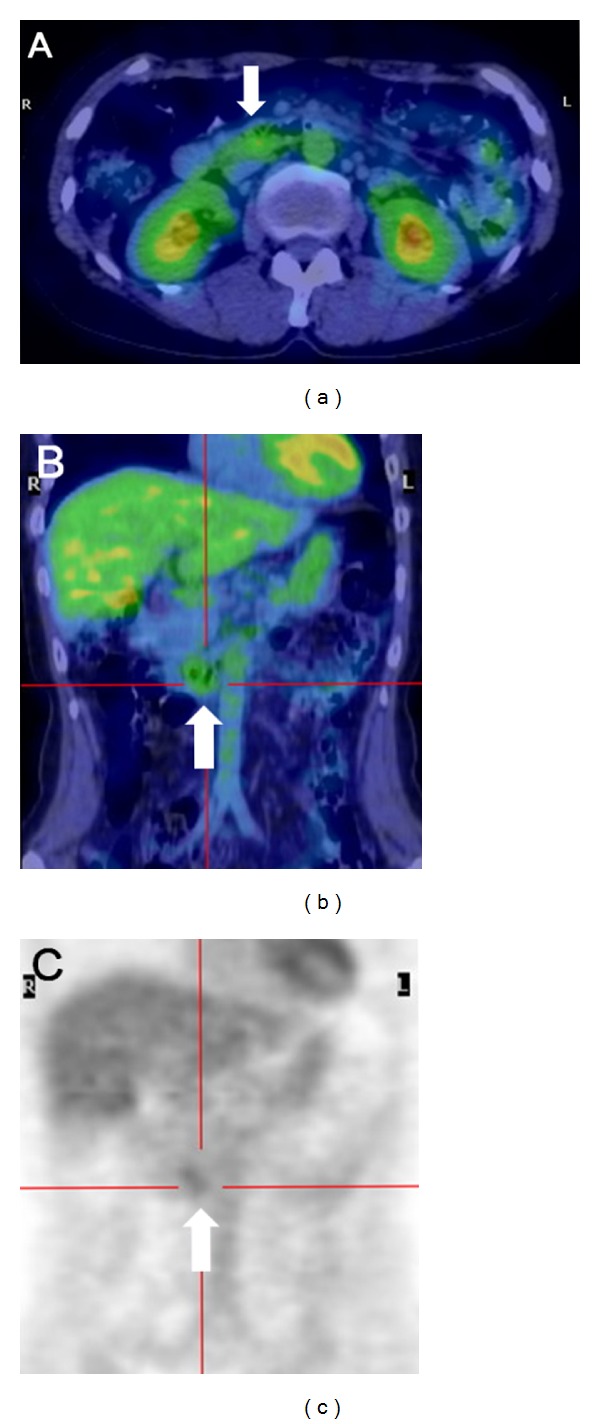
18F-FDG PET images of the patient. Positive tracer uptake in the duodenum was shown in the horizontal section ((a), arrow) and coronal sections ((b, c), arrows). No abnormal uptake in other organs including the lymph nodes was detected.
